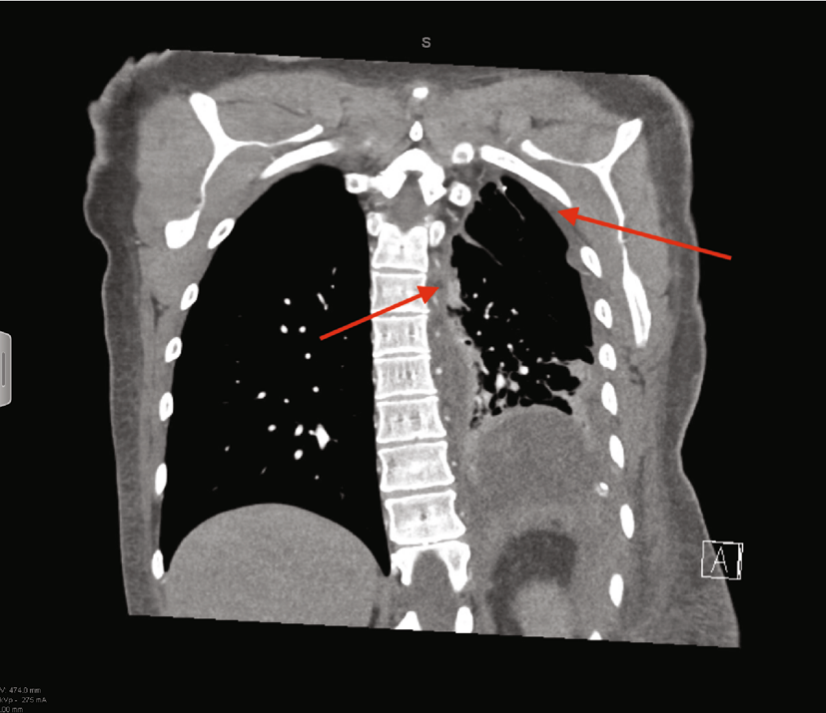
CT of the thorax (coronal). Diffuse nodular thickening of the left pleura with mediastinal lymphadenopathy.
